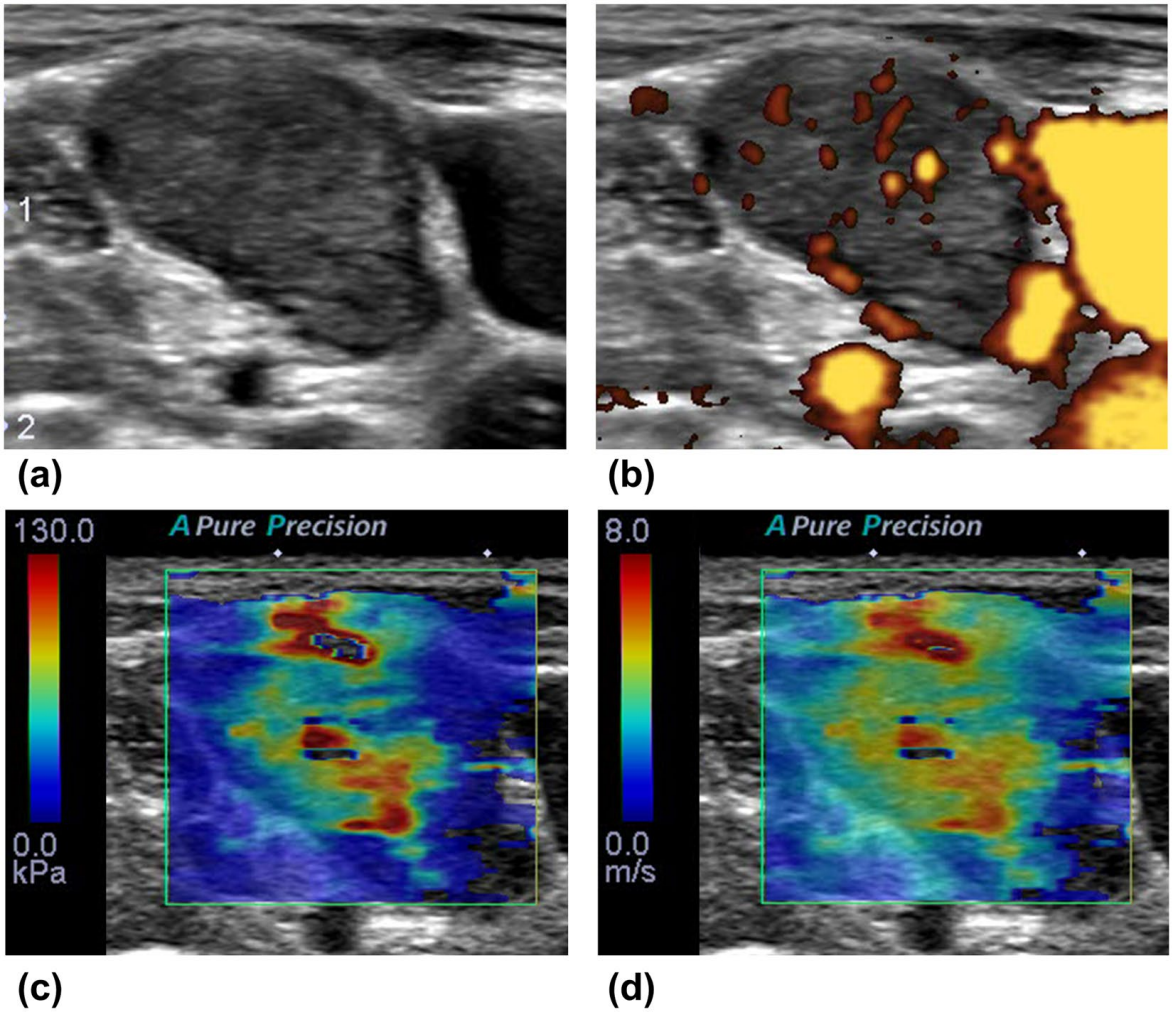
The case of metastatic squamous cell from lung. On conventional ultrasound (a: 2D echo, b: power Doppler US), an oval lymph node with loss of hilum has relatively homogeneous high internal echogenicity and shows regular border with internal mixed vascularity. Two readers diagnosed these lymph nodes as lymphoma with 2D and Doppler US. After evaluation with shear wave elastography (c: the elasticity map, d: the speed map), all readers classified the nodes as pattern 3, and the two readers corrected their diagnosis as metastasis. Maximum elasticity, relative elasticity, maximum speed and relative speed were 92.3 kPa, 7.1, 7 m/s and 3.44, respectively.
